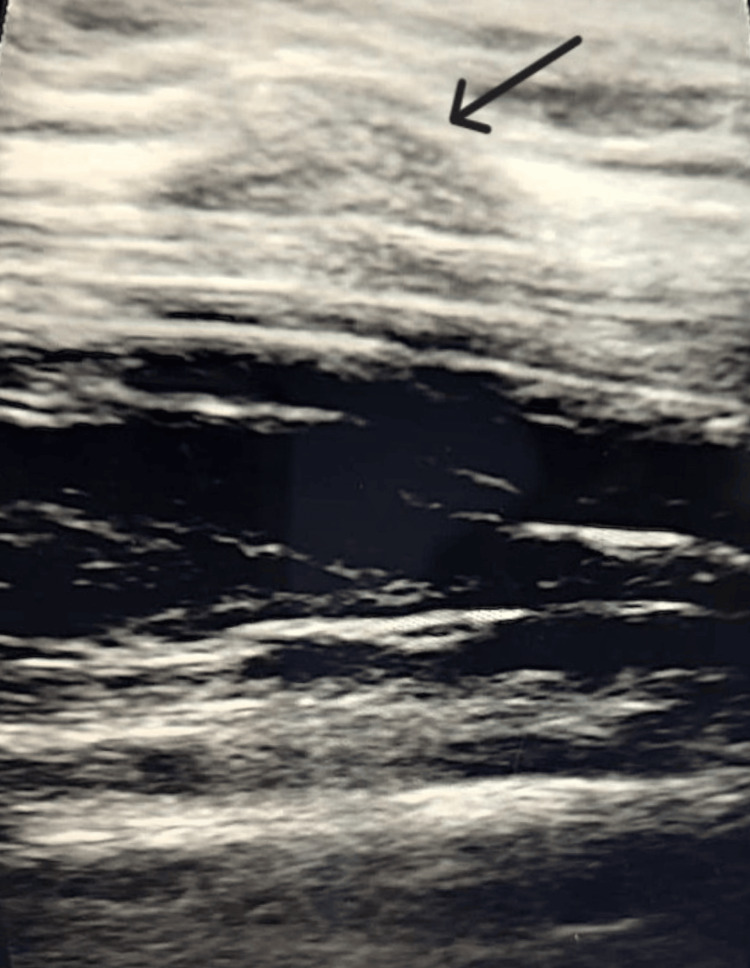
Dynamic ultrasonography demonstrating focal loss of fascial continuity with protrusion of the tibialis anterior muscle during contraction. The black arrow indicates the herniated portion of the tibialis anterior muscle protruding through the fascial defect.
